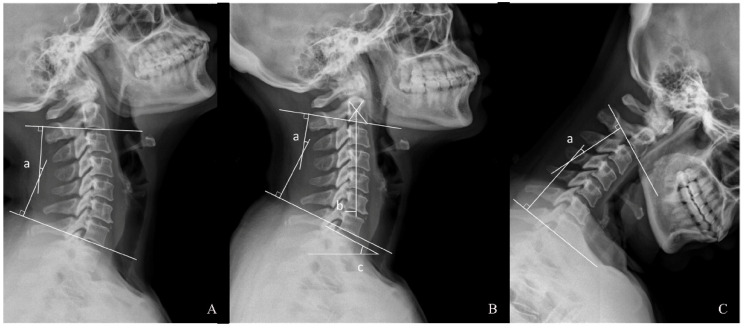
Radiographic measurements: (A) extension, (B) cervical lateral and (C) flexion radiographs, a. C2–7 Cobb angle, b. C2–7 sagittal vertical axis (SVA) and c. T1 Slope (T1S).
